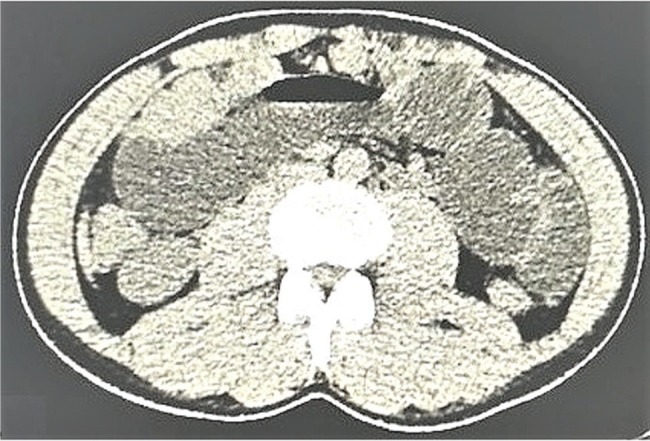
Computed tomography showing a suspected mass-mimicking lesion in the right kidney in a 55-year-old man with clear cell renal carcinoma.
